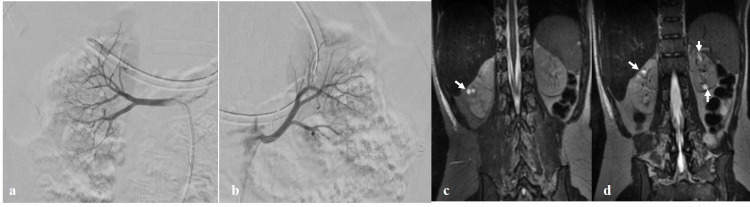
Catheter angiogram of bilateral renal arteries and MRI abdomen. The diagnostic catheter angiogram of bilateral renal arteries (right renal artery (a) and left renal artery (b)) does not reveal any obvious narrowing of renal arteries or their segmental branches. Coronal T2 fat-saturated images of abdomen (c, d) show few cysts within both the kidneys (white arrows). The left kidney is relatively smaller in size (left kidney: 7 cm and right kidney: 8.3 cm).
